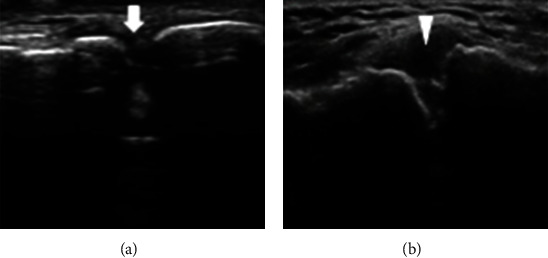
Ultrasound images of A-C joint in healthy (a) and painful (b) shoulders. A-C joint in healthy shoulder (arrow). In A-C joint degeneration and sprain, joint hyperosteogeny and capsular swelling can be observed (arrowhead).
